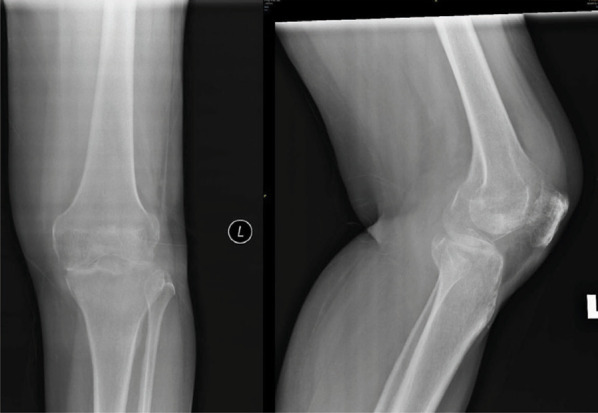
Radiographs AP and lateral views of the left knee, on admission show erosion of the medial tibial and femoral condyle with reduced joint space.
